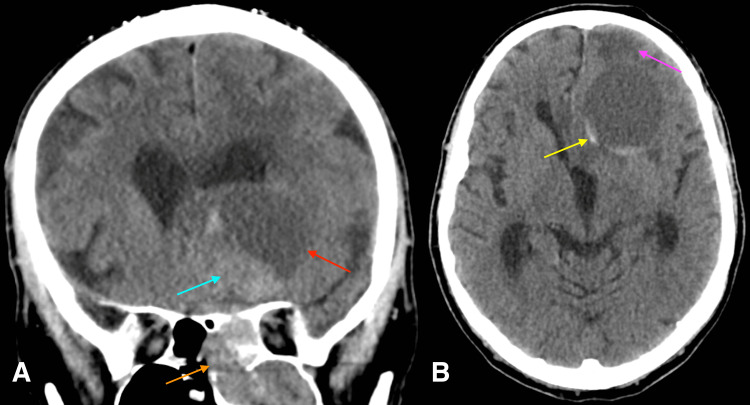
Computed tomography of the head without contrast in the coronal (A) and axial plane (B) Images demonstrate soft tissue opacification involving the left ethmoid and maxillary sinus (orange arrow), with extension into the anterior cranial fossa, where there is lobulated soft tissue (blue arrow) and a cystic component (red arrow); moderate vasogenic edema involving the frontal lobe anteriorly (pink arrow); and some peripheral hyperdensity associated with the cystic component of the lesion (yellow arrow), which could represent a small amount of blood product in the dependent aspect
